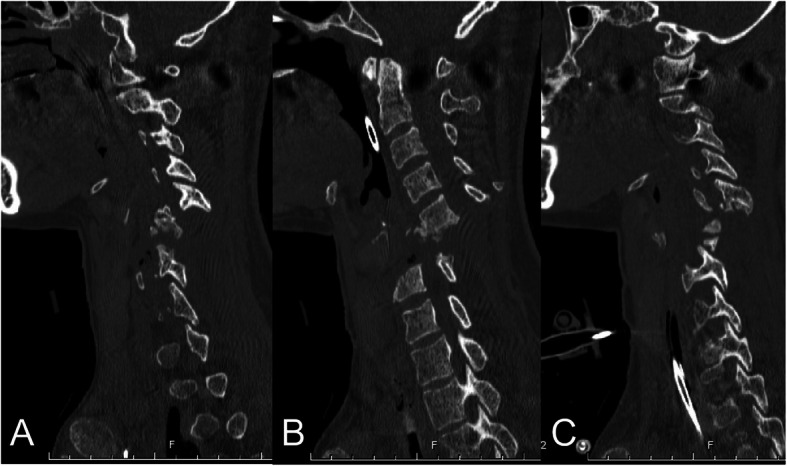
CT films showed fracture dislocation at C5-6 levels with significant widen at C5-6 intervertebral space
